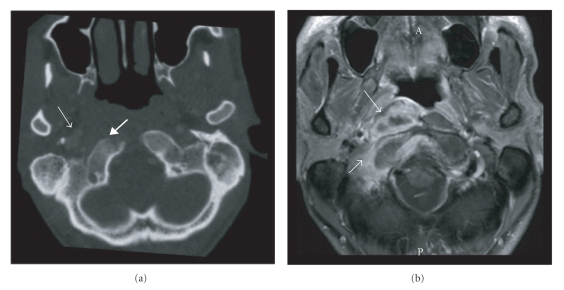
(Case B) Axial contrast-enhanced CT image in bone window (a) and axial T1-weighted MR image with fat suppression after administration of Gadolinium (b) at the level of the foramen magnum. Indicated in (a) are the mass on the right side of the nasopharynx (medium open arrow) and lysis of the clivus (medium closed arrow). The abscess in the retropharyngeal space and prevertebral muscle is better appreciated on MR (b, medium open arrow); edema in the clivus and occipital bone as well as paravertebral soft tissues are also indicated (short open arrow).
